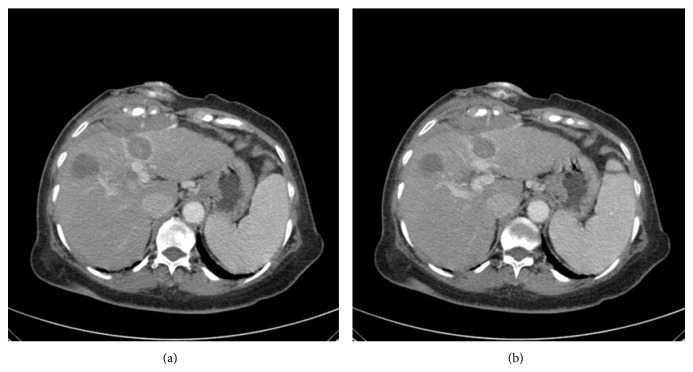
A dual-phase dynamic contrast-enhanced CT was performed just after the RFA procedure which revealed a jet-like extravasation from the intercostal vessels.
